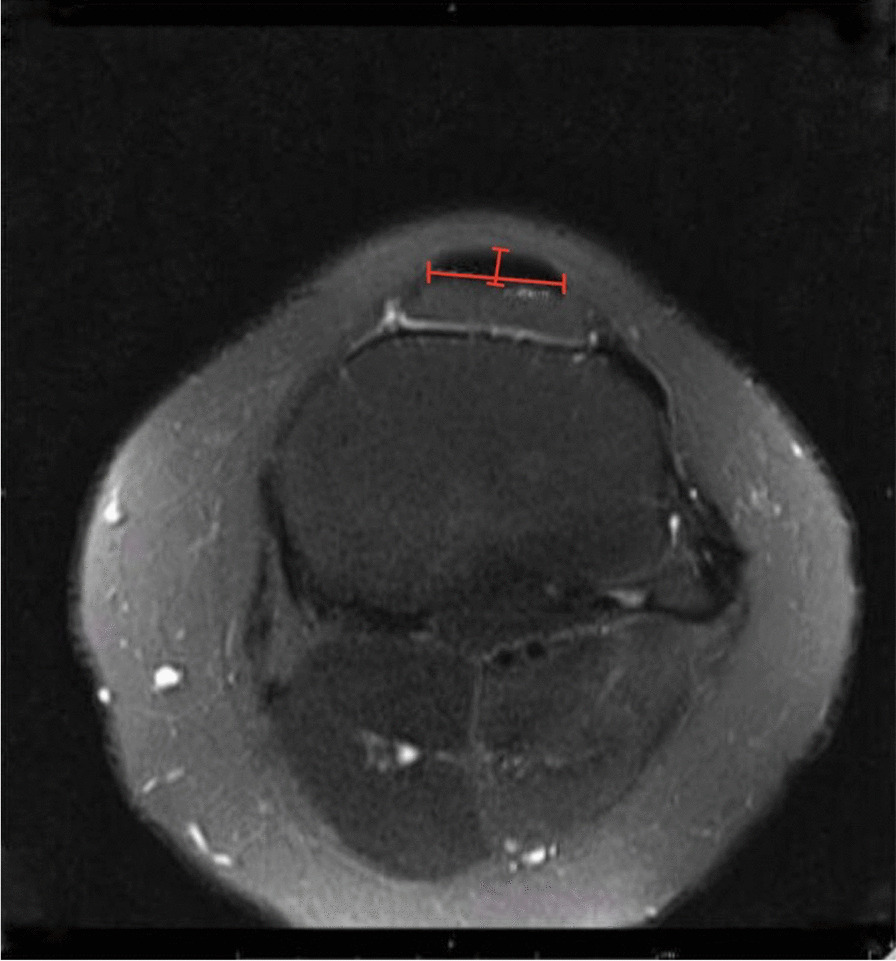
The measurement of patellar tendon horizontal and vertical length on a T2 axial view magnetic resonance image
